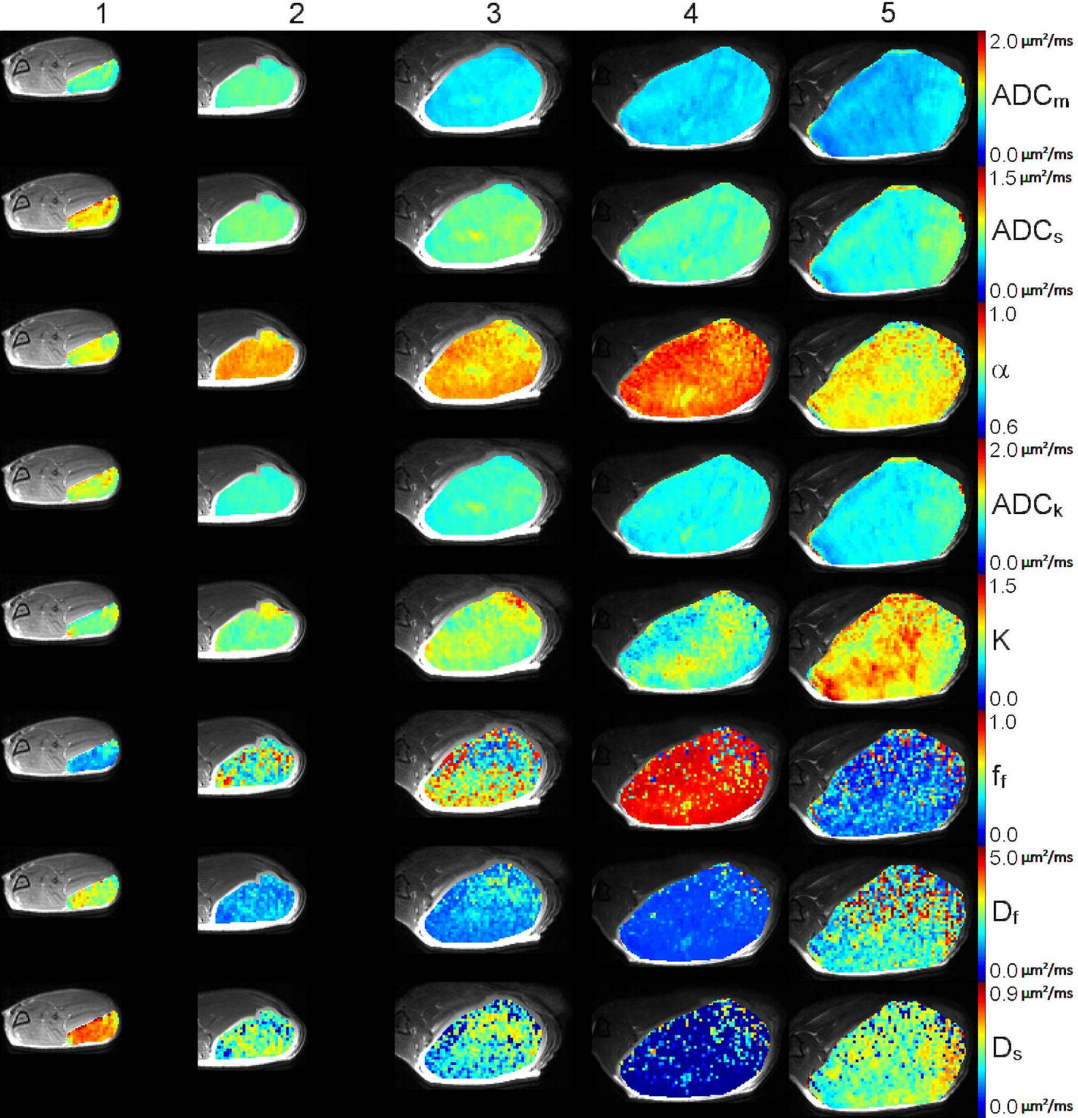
High b-value DWI data of a representative tumor: T2-weighted image fused with parametric maps for ADC_m,_ ADC_s_, α, ADC_k_, K, f_f_, D_f_, and D_s_ parameters are shown, and represent different degree of tumor homogeneity between week 1 (column 1), week 2 (column 2), week 3 (column 3), week 4 (column 4). Furthermore, the second repetition done on week 4 is shown (column 5). The parametric maps are scaled as follows: ADC_m_ (A1−5) min−max: 0−2.0 µm^2^/ms, ADC_s_ (B1−5) min−max: 0−1.5 µm^2^/ms, α (C1-5) min−max: 0.6−1.0, ADC_k_ (D1−5) min−max: 0−2.0 µm^2^/ms, K (E1−5), min−max: 0−1.5, f_f_ (F1−5), min−max: 0−1.0, D_f_ (G1−5) min−max: 0−5.0 µm^2^/ms, D_s_ (H1−5) min−max: 0−0.9 µm^2^/ms.
